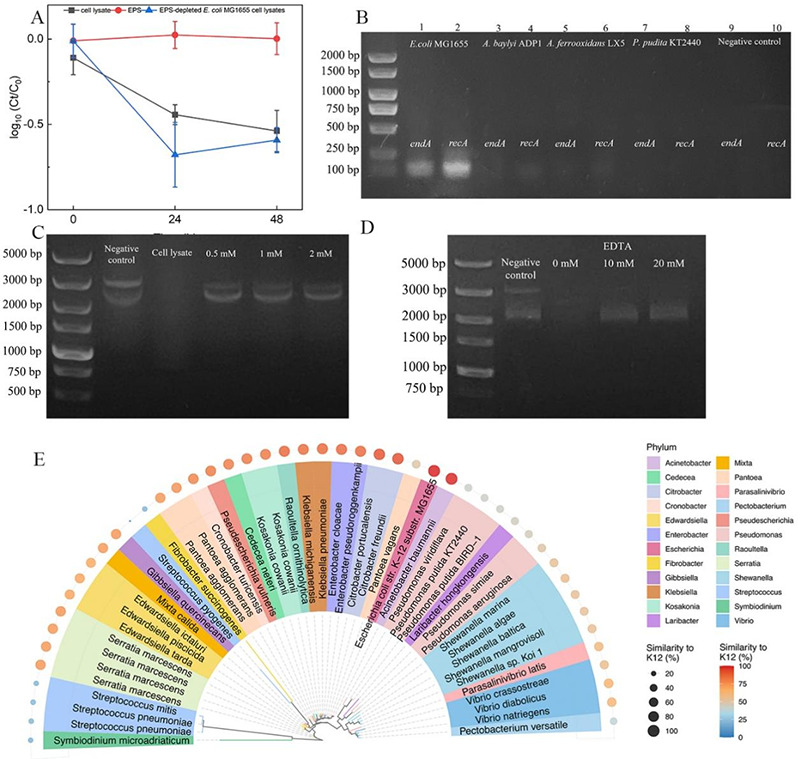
Evidence of eARG degradation by existing DNase in the cell lysate of *E. coli* MG1655. (A) Changes in the logarithm of eARG copies degraded by the cell lysate, EPS fraction, and EPS-depleted *E. coli* MG1655 cell lysates. Cell lysate, soluble EPS, and EPS-depleted *E. coli* MG1655 cell lysates were prepared by ultrasonic splitting of 10^9^ cells/mL of *E. coli* MG1655 (WT). (B) Identification of the *endA* and *recA* genes in *E. coli* MG1655, *A. baylyi* ADP1, *A. ferrooxidans* LX5, and *P. putida* by agarose gel electrophoresis. (C) Gel electrophoresis of plasmid pUC19 after 48 h of co-incubation with cell lysate or cell lysate spiked with different concentrations of a nuclease inhibitor. (band 1: negative control; band 2: co-incubation with cell lysate (0.24 mg/L); band 3–5: co-incubation with cell lysate (0.24 mg/L) spiked with different concentrations of nuclease inhibitor (0.5, 1, and 2 mM). (D) Gel electrophoresis of plasmid pUC19 after 48 h of co-incubation with cell lysate (0.24 mg/mL) or the cell lysate spiked with different concentrations of EDTA (10 mM and 20 mM) (band 1: negative control; band 2: co-incubation with cell lysate; band 3–4: co-incubation with cell lysate spiked with different concentrations of EDTA (10 mM and 20 mM) to assess the degradation of plasmid pUC19. (E) The distribution of protein *endA* in the selected bacteria (shown in phylogenetic tree). Matching scores reflect the amino acid sequence identity of the EndA protein, similarity between *E. coli* MG1655 and other bacteria. The larger circular legend represents greater similarity.
